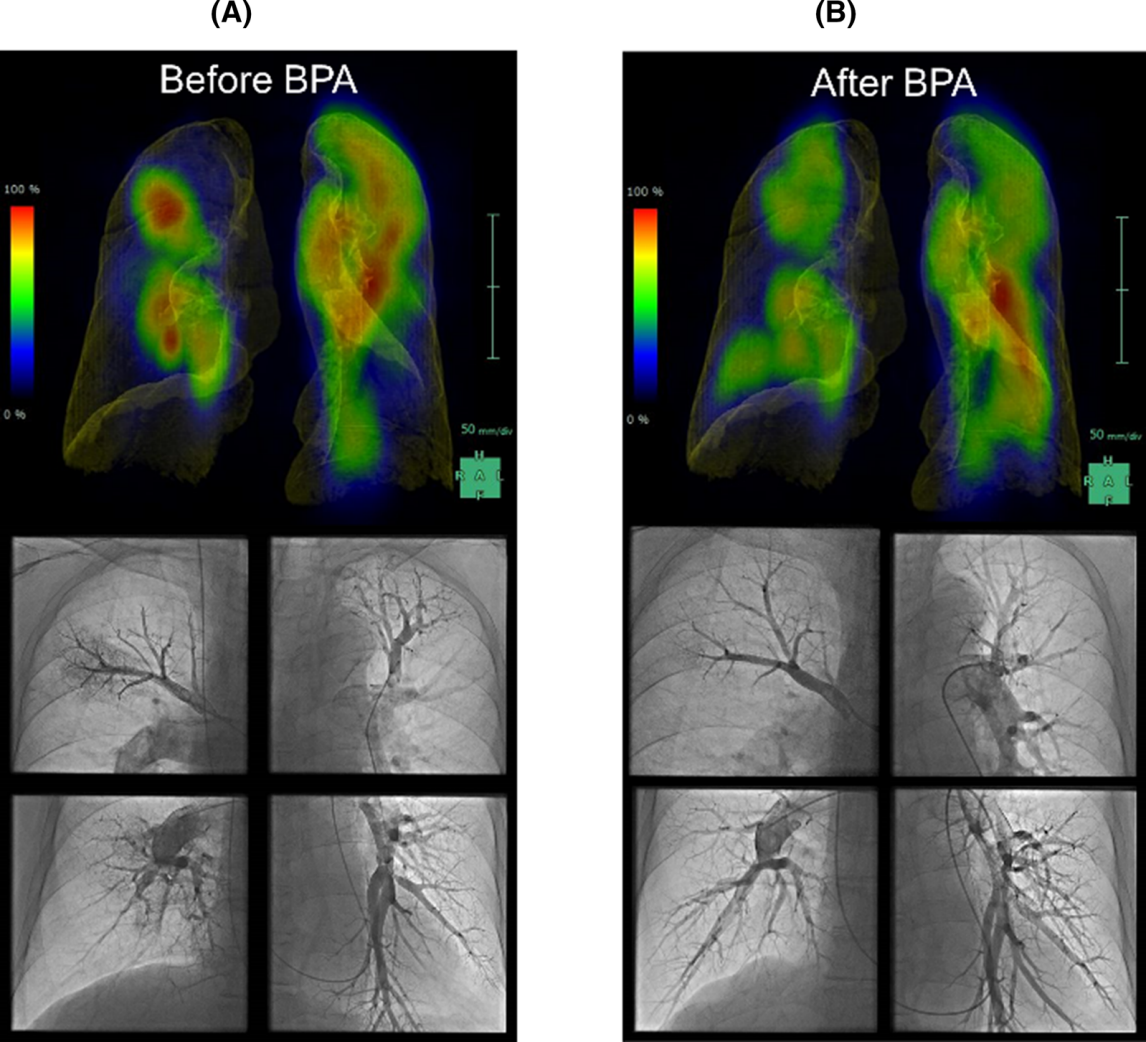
SPECT and CT fusion images and pulmonary artery angiographic images of a patient with CTEPH before and after BPA. SPECT, single-photon emission computed tomography; CT, computed tomography; CTEPH, chronic thromboembolic pulmonary hypertension; BPA, balloon pulmonary angioplasty
